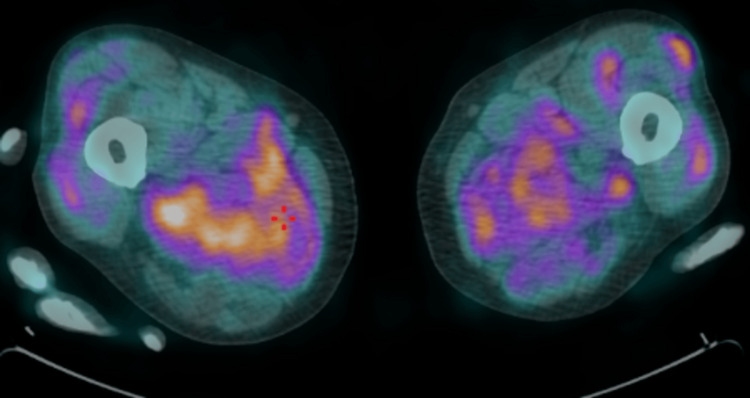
“Tiger man sign" on 18F-FDG PET/CT (axial fused image at the thigh level), demonstrating diffuse, heterogeneous, and fascicular-pattern FDG uptake in the skeletal muscles of both lower limbs. FDG: fluorodeoxyglucose.
